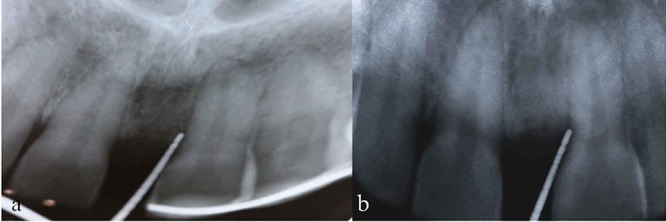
a: Post-operative radiographic view of IOPA at 3rd month  b: Post-operative radiographic view of IOPA at 6th month
